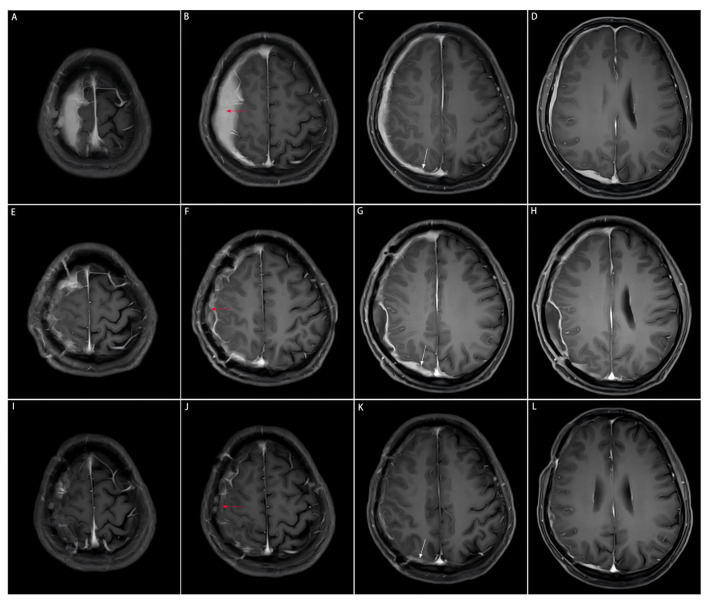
Comparison of preoperative, postoperative, and post-medication enhanced-contrast MRI images. The area of the resected dural mass before and after the subtotal resection, and after medication on enhanced-contrast MRI images is indicated by the red arrow. The dural mass close to the cerebral falx on enhanced-contrast MRI images is indicated by the white arrow. This part is not surgically removed due to the tricky localization approach to the midline, but significantly shrinks after the three-month medication of prednisone acetate. (A–D): Enhanced-contrast MRI images preoperatively, (E–H): 2 months postoperatively, (I–L): 3 months of prednisone acetate.
